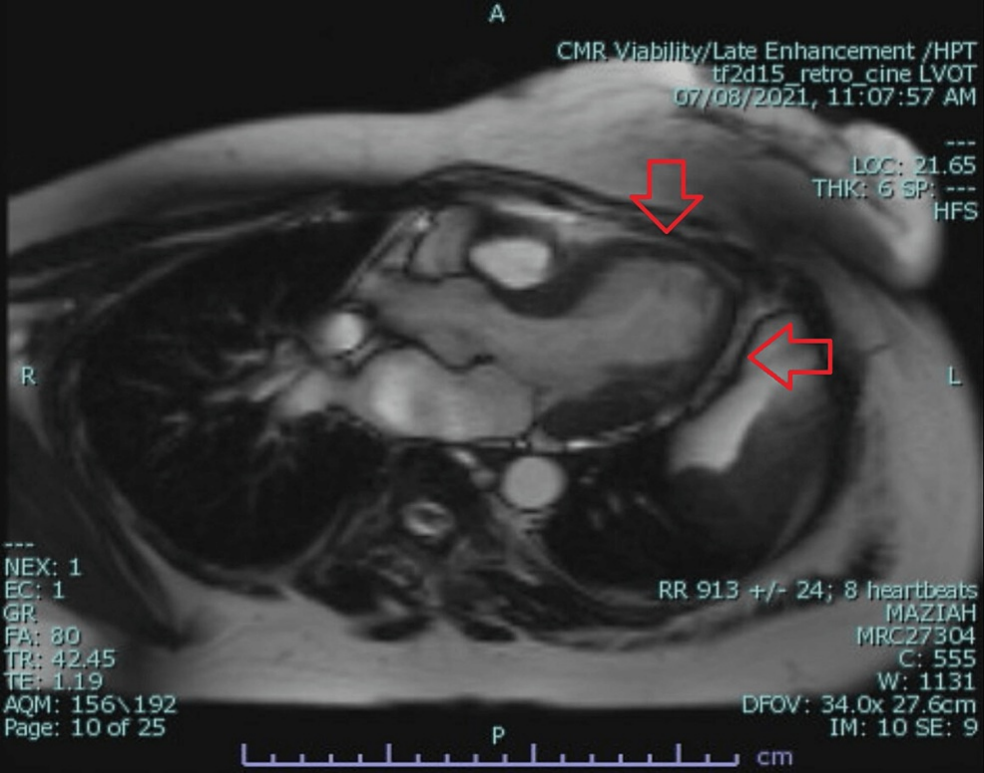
Cardiac magnetic resonance imaging (MRI) showed mid to apical severe hypokinesia with ballooning of the apical segment (red arrow)
